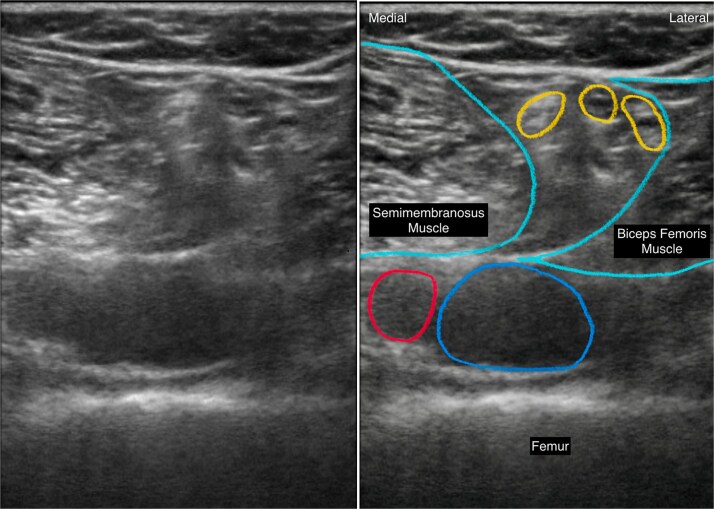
Ultrasound image of the anatomical region targeted in the distal approach to infiltration between the popliteal artery and the capsule of the knee (d-iPACK) block. The ultrasound probe is placed posteriorly over the popliteal fossa, just proximal to the femoral condyles. The popliteal artery (red) and popliteal vein (blue) are highlighted, with muscle borders outlined in cyan. Relevant muscles and bony structures are labeled.
